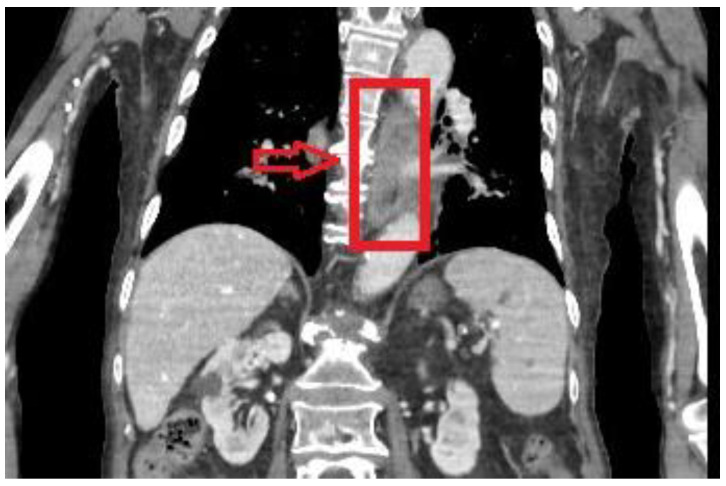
Computed tomography showing perieto-mucosal thickening of the medium–distal tract of the esophagus.
